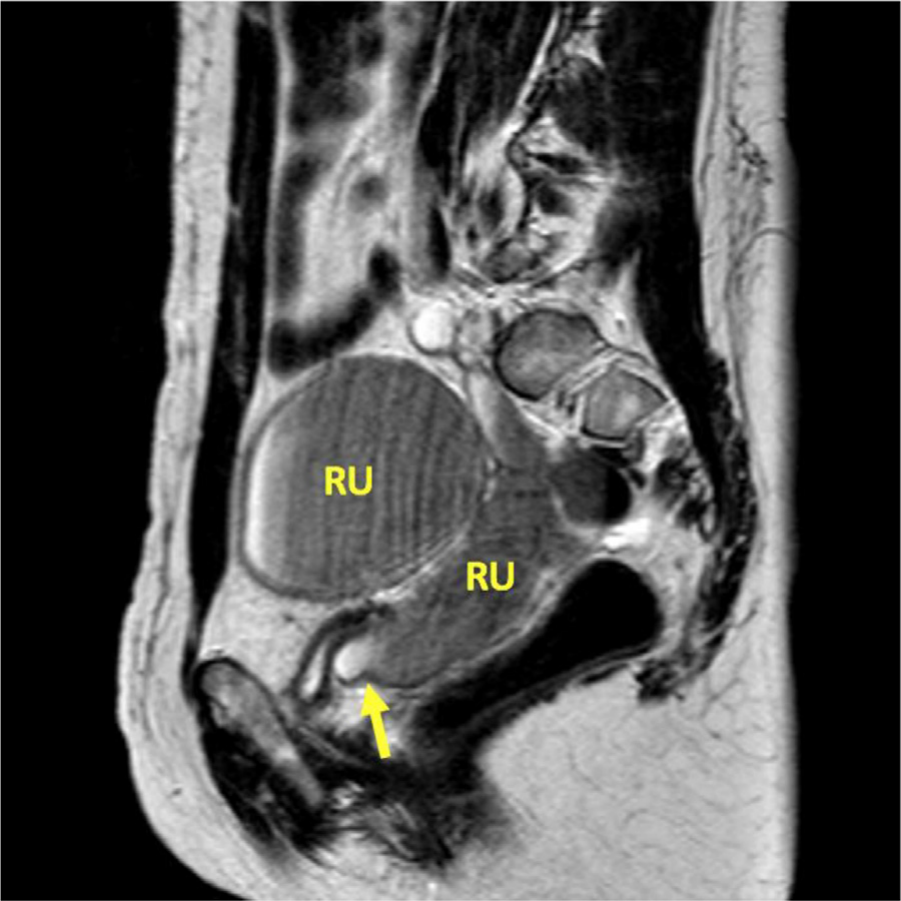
MRI T2-Weighted sagittal image. Transverse membrane (arrow) obstructing the right hemivagina.
